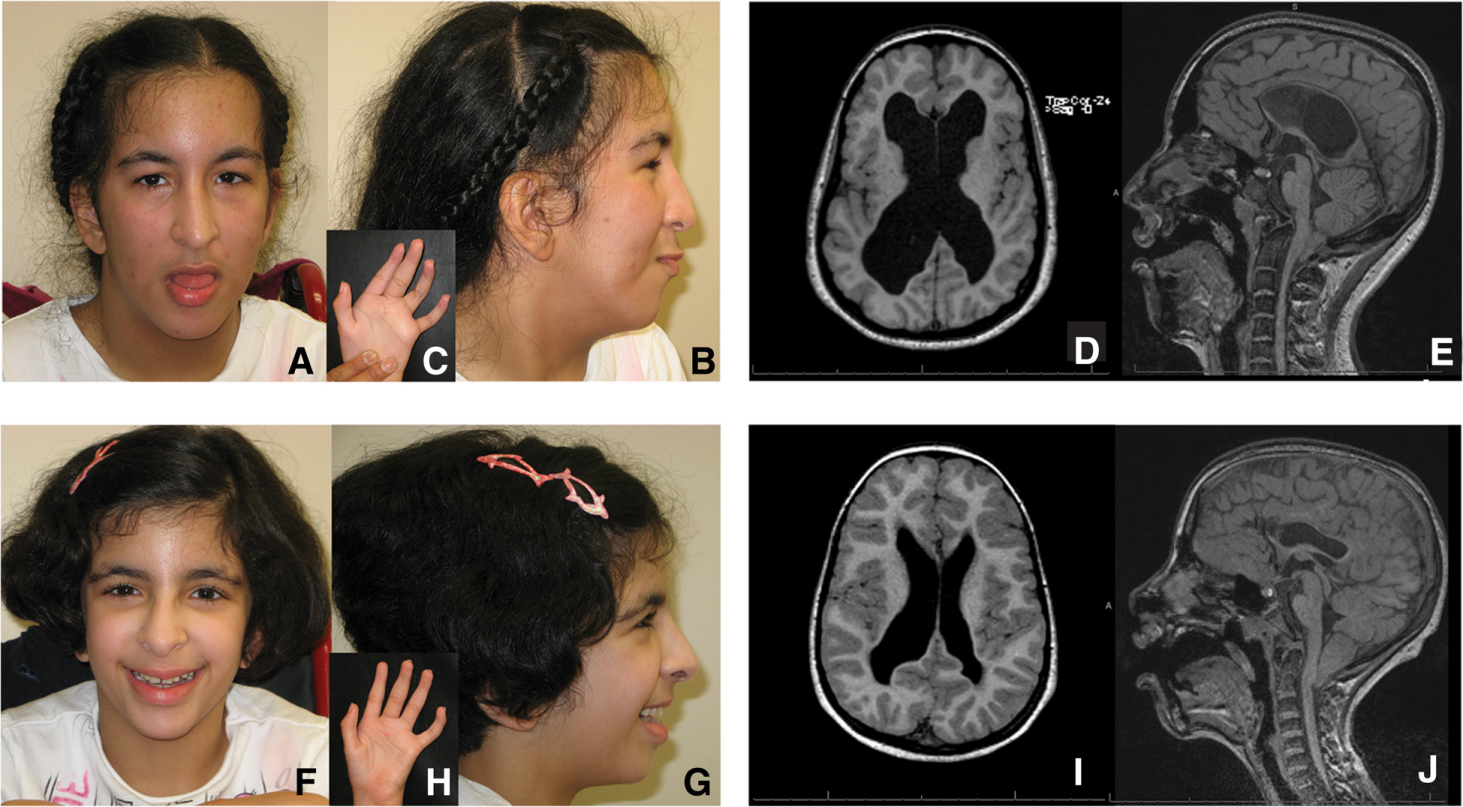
Clinical and MRI features of siblings with Desmosterolosis. A to C: Patient 1 craniofacial and hand features at age 14.8 years. Her dysmorphic features show scaphocephaly, tall forehead with bitemporal narrowing, short palpebral fissures, long nose, hypoplastic nasal alae, prominent columella, and low-set posteriorly rotated ears. D, E (Axial T1, Sagittal FLAIR): Patient 1 brain MRI showing white matter volume loss, dilated ventricles, thin corpus callosum, and peg-like cerebellar tonsils displaced into the upper cervical canal through the foramen magnum (Chiari I malformation). F to H: Patient 2 craniofacial and hand features at age 10.1 years, similar to Patient 1. I, J (Axial T1 and Sagittal FLAIR): Patient 2 brain MRI showing prominent and irregular ventricles, thin corpus callosum, and Chiari I malformation.
